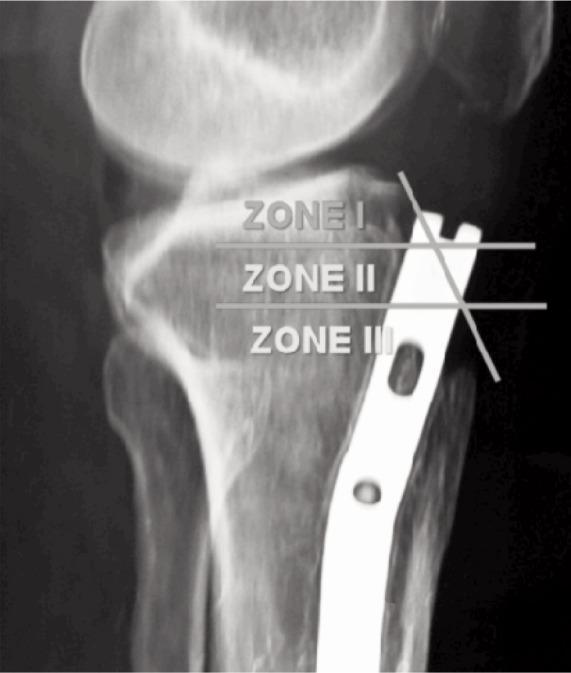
Lateral radiograph showing division of proximal tibia into three zones.
